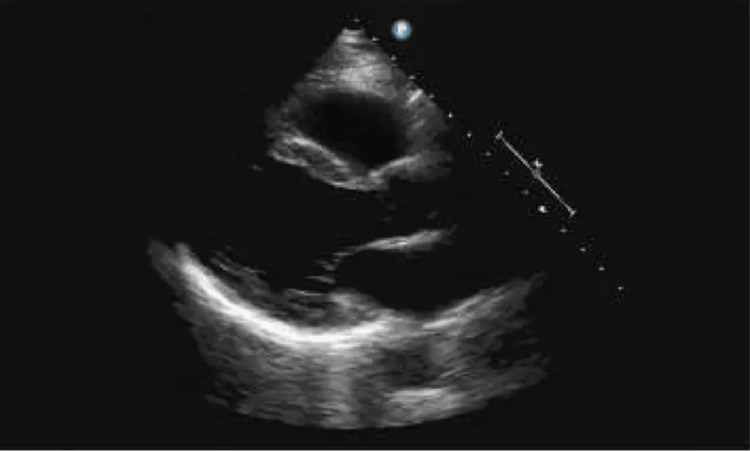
TTE (parasternal long-axis view) demonstrating the left ventricle and mitral valve without evidence of vegetations TTE: transthoracic echocardiogram
